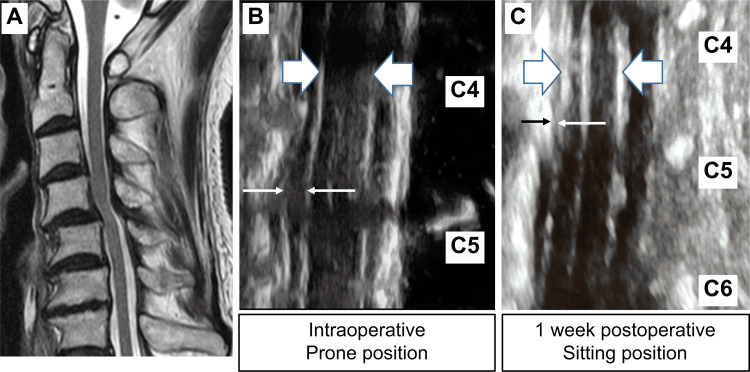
(A) Preoperative MRI, (B) intraoperative ultrasound findings, and (C) one-week postoperative ultrasonography findings of an 80-year-old male, diagnosed with cervical spondylotic myelopathy who underwent laminoplasty. The interior of ➡︎ (thick line) indicates the spinal cord, and the interior of → (thin line) indicates the ventral subarachnoid space. Intraoperatively, the ventral subarachnoid space was continuous, and decompression was grade 1. One week postoperatively, the spinal cord was in contact with the posterior elements of the vertebral body at the C4-5 level, and decompression status was Grade 2. The intraoperative pulsation pattern was pattern 5, “wave motion,”; however, postoperatively, a pattern 1 "pulsation" beat was observed.
